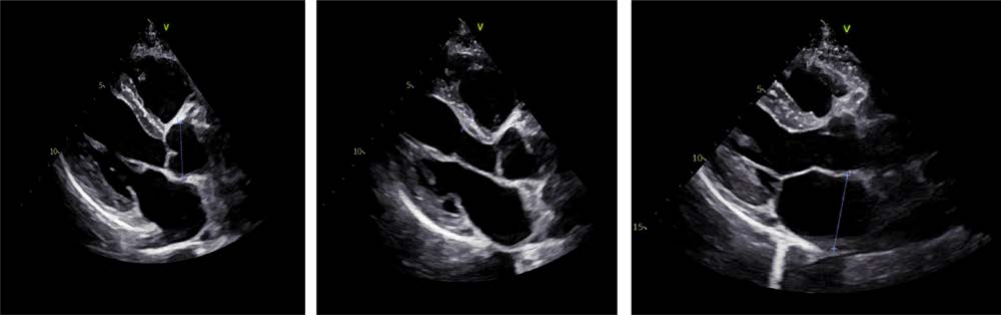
Cardiac ultrasound: cardiac measurements: AO: 32 mm, IVSd: 9 mm, LVDd: 46 mm, LVFS: 41%, LA: 41 mm, LVPWd: 8 mm, LVDs: 27 mm, LVEF: 72%. The left atrium is full, tricuspid regurgitation is slight, and pericardial cavity has few hydrops.
